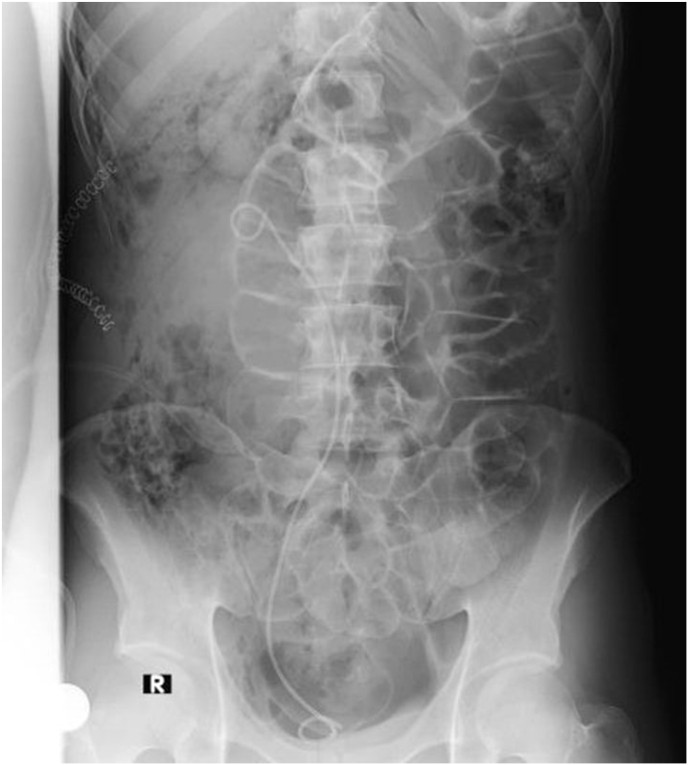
Post-operative renal CT scan showing inserted double J.
